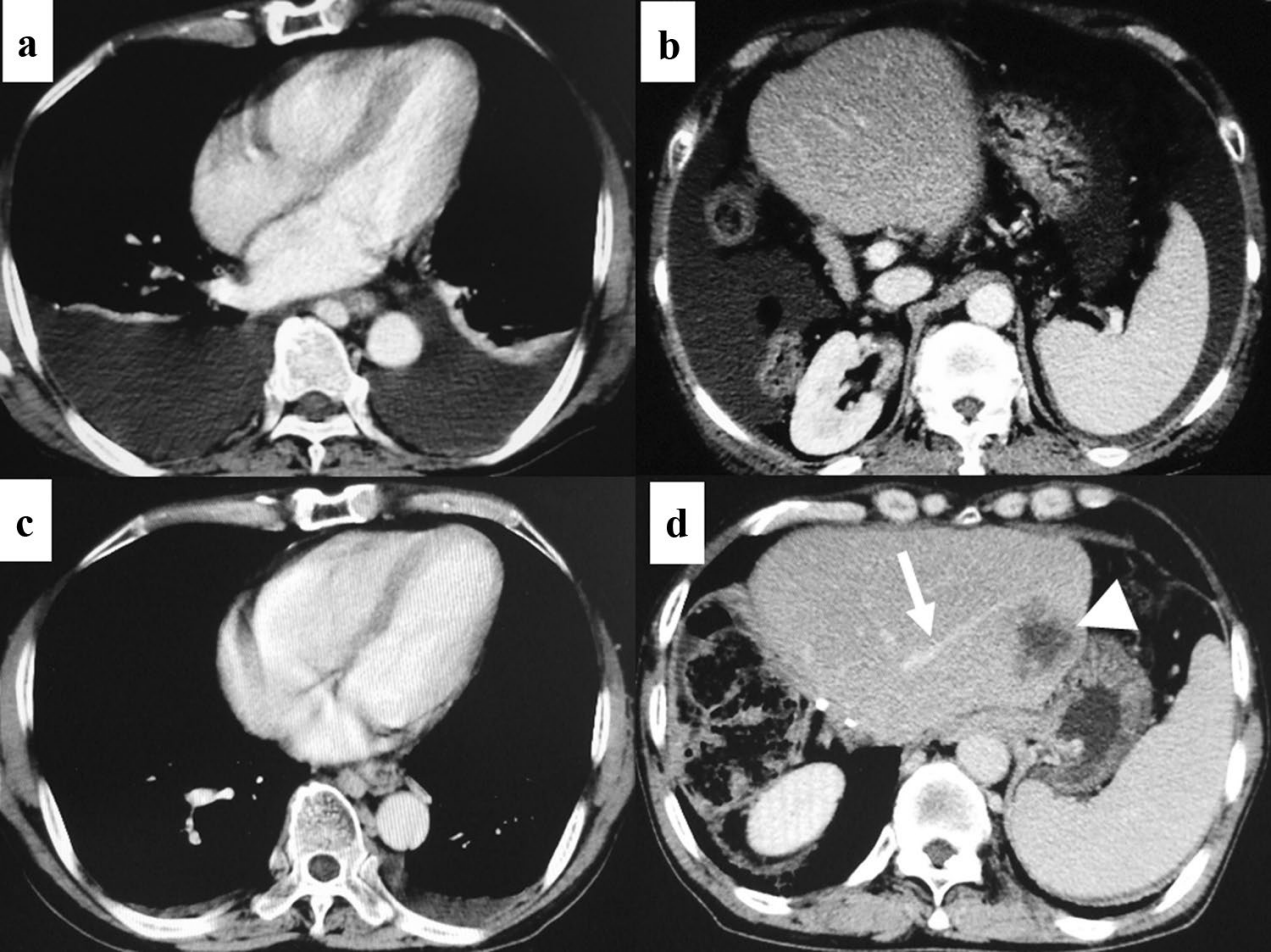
Thoracoabdominal computed tomography findings. Large amount of bilateral pleural effusion (a), massive ascites (b), and splenomegaly (b) were observed in the thoracoabdominal computed tomography taken at the transfer to our institution. Four weeks after initiating the use of tolvaptan, however, pleural effusion (c) and ascites (d) were almost entirely eradicated. Splenomegaly remained (d). A relatively large tumor (white arrowhead, d) was observed near the left hepatic vein (white arrow, d) in the remaining liver
